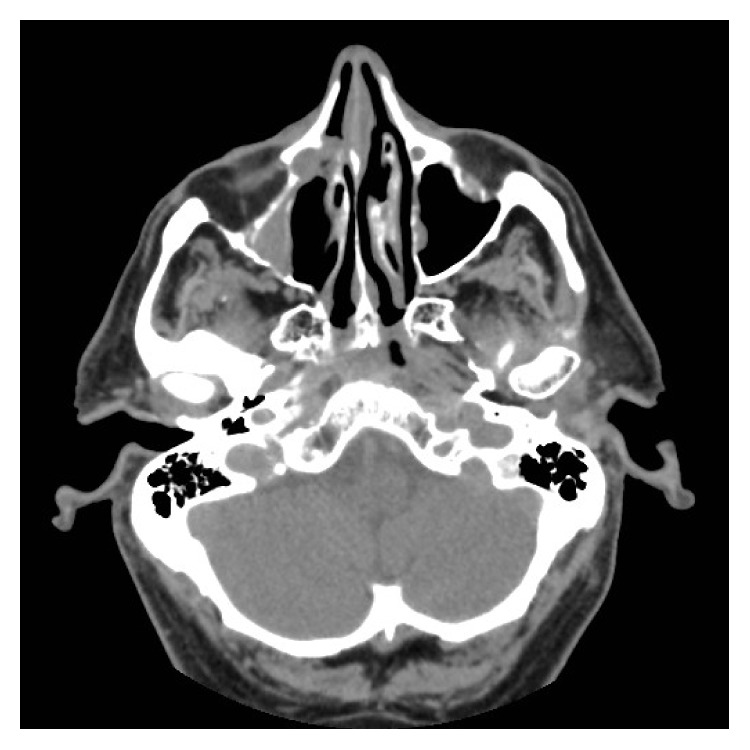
CT sinuses: axial section showing the lesion within the right nasolacrimal duct with evidence of bony remodelling.
